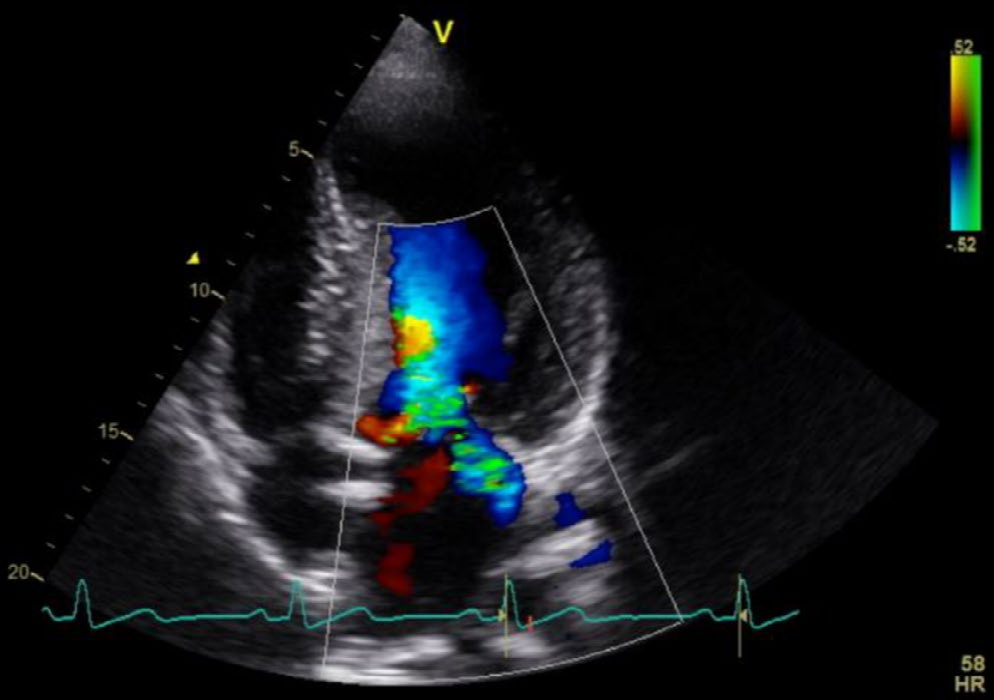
Transthoracic color duplex echocardiography of the heart in the apical three‐chamber view showing a regurgitant jet into the left atrium, consistent with mitral insufficiency, Grade 3.
